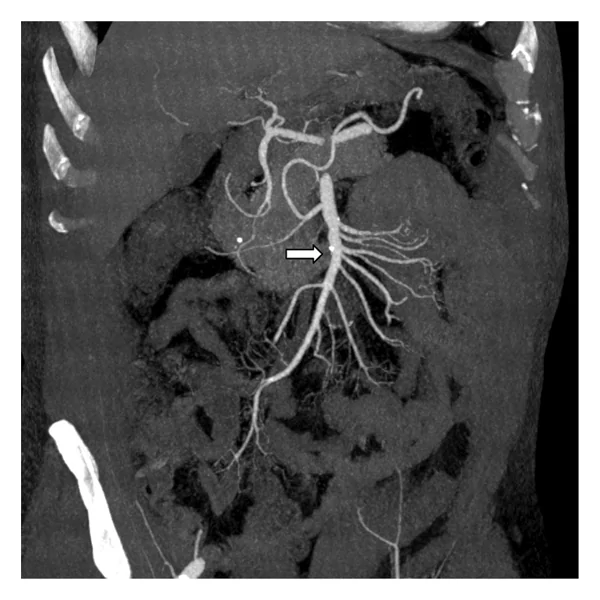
The coronal MIP view from a contrast‐enhanced abdominal CT angiography highlighting the calcific plaques located in the middle section of the SMA. This projection provides a clear view of plaque distribution along the artery, which can lead to stenosis and impair blood flow, potentially resulting in mesenteric ischemia.
